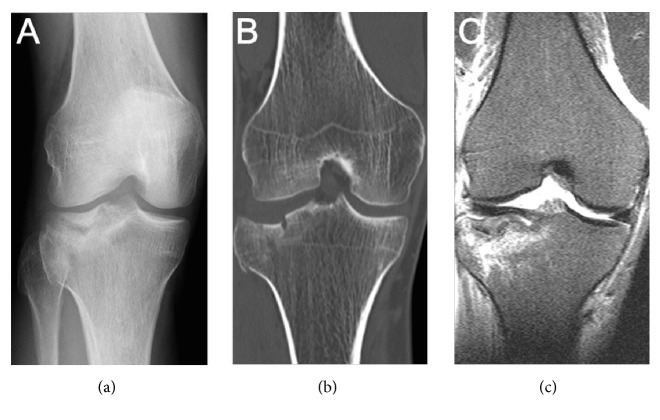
A 33.6-year-old female presented with a right lateral tibial plateau fracture. (a) Anteroposterior radiograph of the injured knee, (b) CT demonstrated a Schatzker II fracture with a maximum lateral plateau widening of 9 mm (coronal CT scan), and (c) MRI with a lateral collateral ligament lesion.
